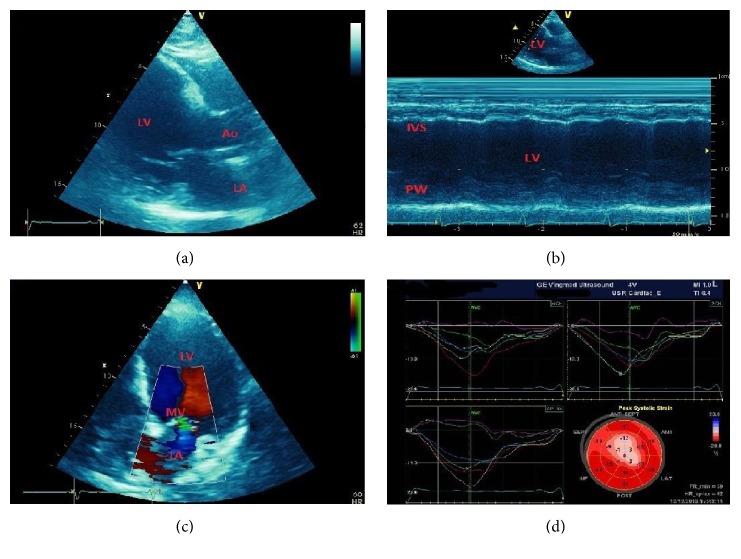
A 43-year-old male patient with anteroapical myocardial infarction. Subsequent stent implantation in the left anterior descending (LAD) coronary artery and medical therapy. (a) Dilated left ventricle (LV) in long axis view. (b) Akinetic scarred septum (IVS) in M-mode representation. (c) Dilated LV in apical 4-chamber view with mitral valve (MV) regurgitation. (d) White scarred segments compared to red normokinetic segments in the “bull's-eye” plot by speckle-tracking echocardiography. Strain curves show reduction of anteroapical longitudinal strain. Ao = aorta; LA = left atrium; PW = LV posterior wall.
